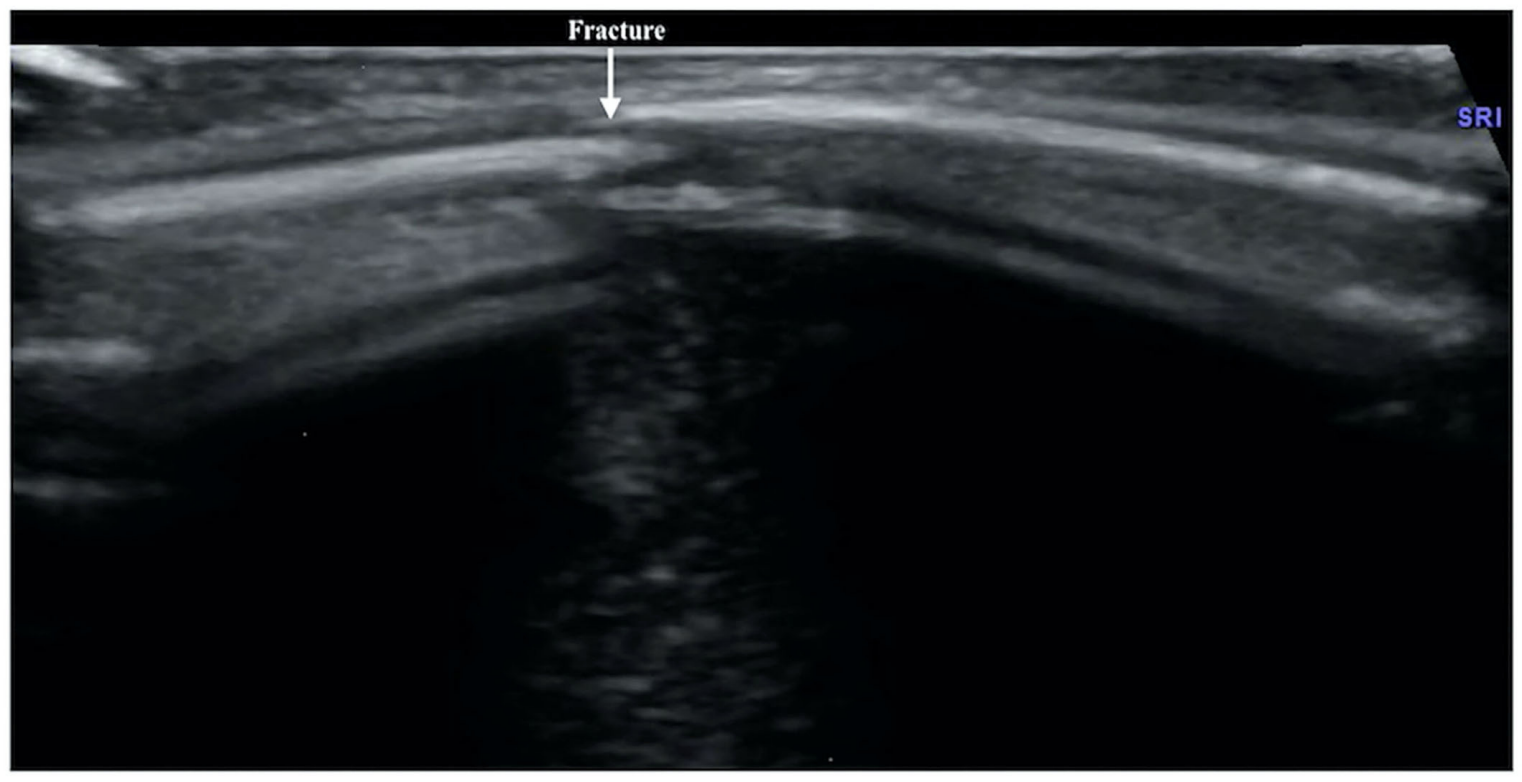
Rib fracture. The infant was G_3_P_1_, with a gestational age of 30^+1^ weeks and a birth weight of 1,370 g. The infant was born by Cesarean section due to placental abruption. After birth, the infant suffered from various diseases, such as respiratory distress syndrome, pneumonia, atelectasis, and calcium and phosphorus metabolism disorder, which was diagnosed as metabolic bone disease. Forty days after birth, an ultrasound examination found that the infant had a fracture in the fifth rib on the left side.
